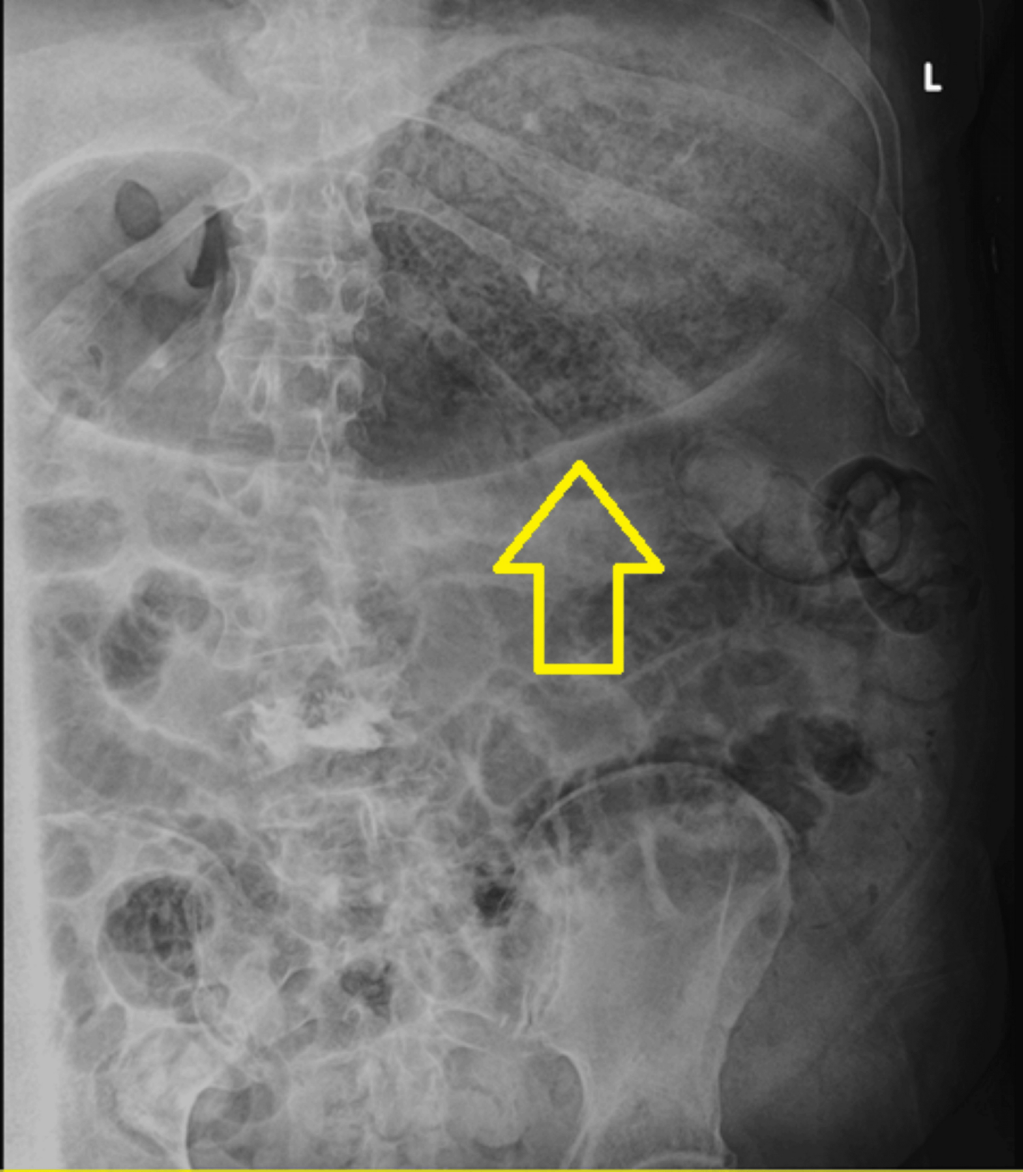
Abdominal radiograph demonstrating gastric distention The arrow indicates the gastric distention.
